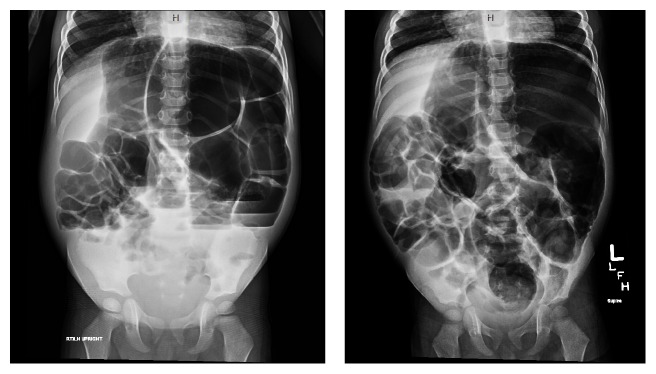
Supine and upright plain X-rays of the patient presented in Case 2. The stomach is markedly distended and filled with air. There is moderate and severe distention of several bowel loops, with air-fluid levels.
